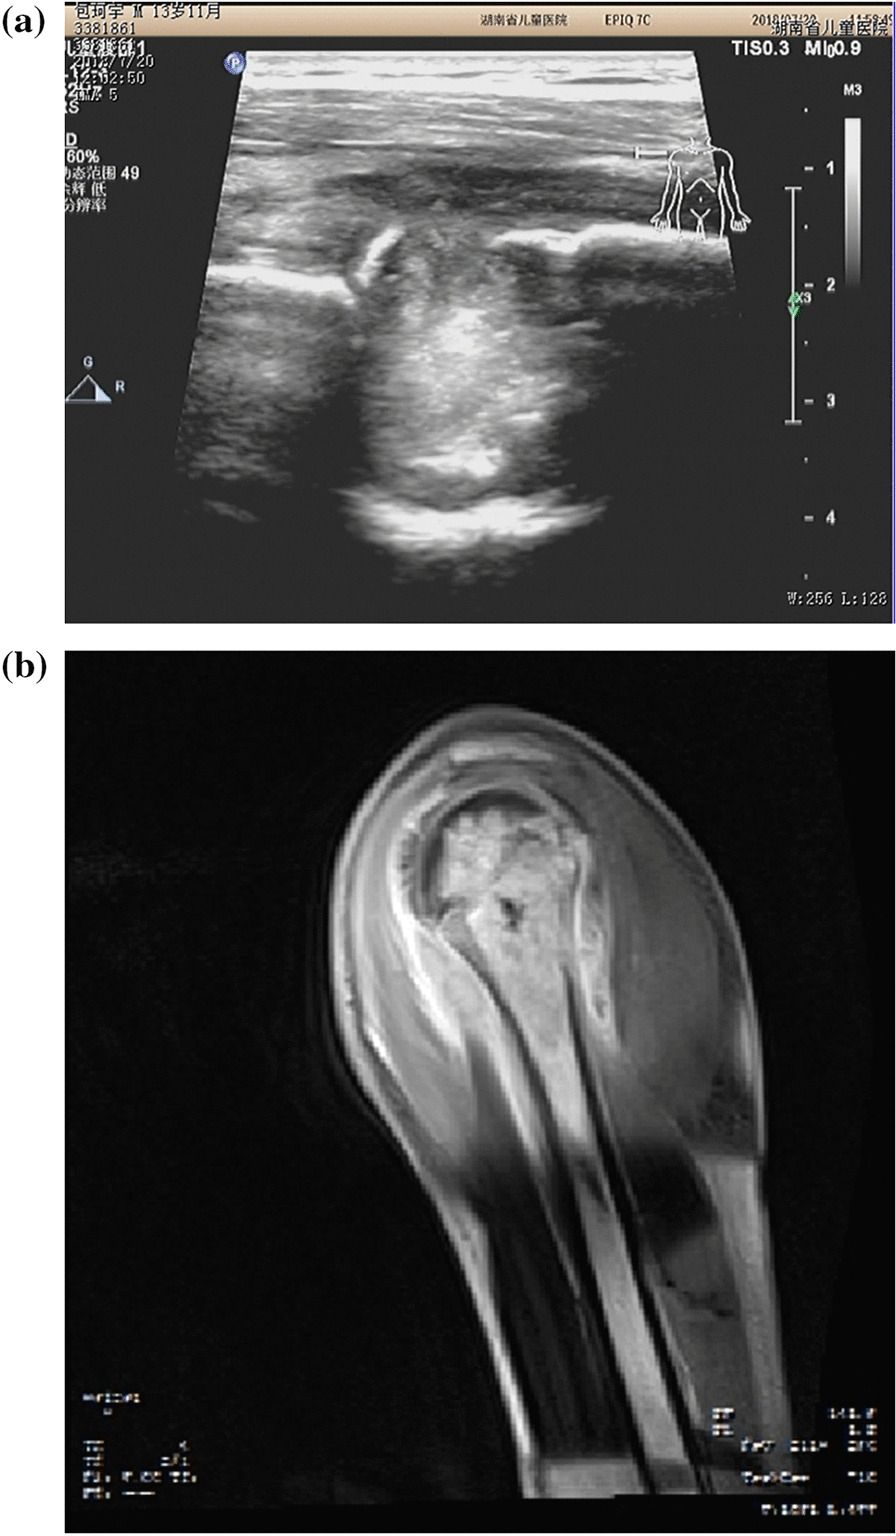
A Bone destruction was severe in the epiphysis, local bone invagination and bone marrow abscess cavity were formed, MRI (B) showed similar findings (f)
